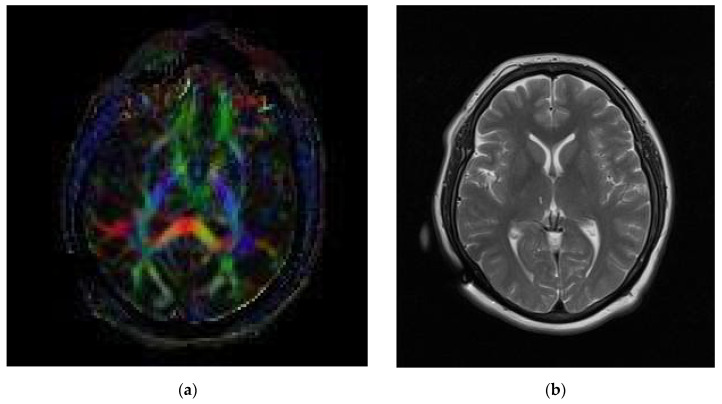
(a) A DTI showing tracts surrounding the left centro-median lesion. (b) Postoperative T2 MRI sequence showing a rounded lesion at the centro-median nucleus 6 months after the procedure.
